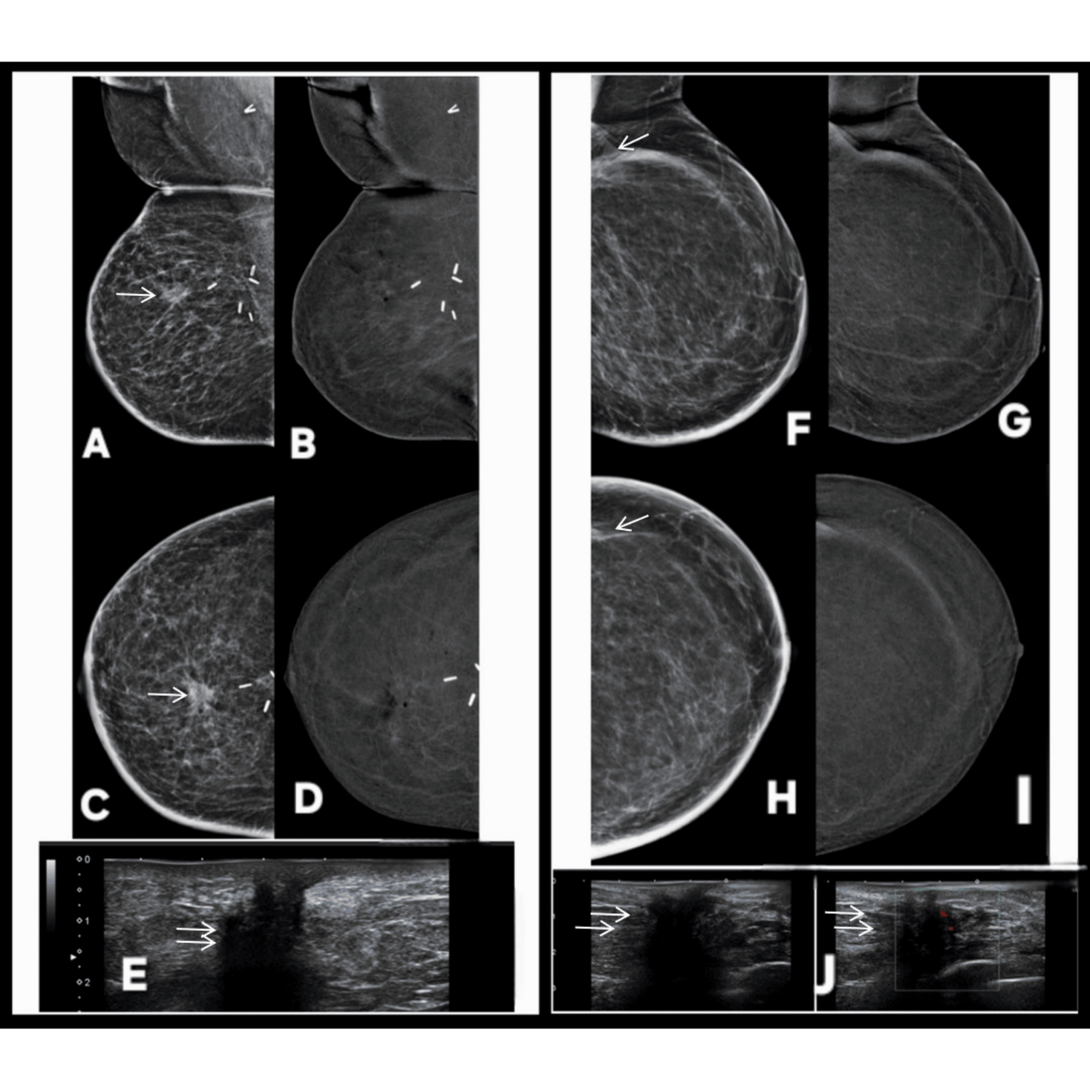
Contrast-enhanced mammography (CEM) as a problem-solving tool in post-surgical architectural distortion: two cases. Described abnormality is annotated by white arrows in all radiological images. Patient ε (Panels A–E): A 50-year-old woman, a three-year post–breast-conserving surgery case for invasive lobular carcinoma, presented for routine surveillance with no available prior imaging for comparison. Digital mammography (DM) in MLO and CC projections (A, C) demonstrates post-surgical clips and an ill-defined area of architectural distortion in the upper inner quadrant (white arrows). Given the oncologic history and absence of priors, the finding was categorized as BI-RADS 3. CEM (B, D) shows complete absence of contrast enhancement at the site of mammographic concern, effectively downgrading the abnormality to BI-RADS 2. Targeted grayscale ultrasound (E) reveals ill-defined posterior acoustic shadowing deep to surgical scar, initially appearing suspicious. However, due to non-enhancing CEM, the finding was reclassified as likely benign post surgical change. At six-month follow-up, stable sonographic appearance is seen, confirming benignity. Patient ζ (Panels F–J): a 55-year-old woman, six-month post–breast-conserving surgery case, was undergoing early routine follow-up. DM images (F, H) show ill-defined area of architectural distortion in the upper outer quadrant (white arrows), suspicious in appearance (BI-RADS 4). Grayscale ultrasound (J) shows a poorly marginated hypoechoic area without definable mass, likewise requiring additional evaluation. CEM (G, I), however, shows no enhancing mass or non-mass lesion, indicating absence of active pathological enhancement and supporting a benign postoperative etiology (BI-RADS 2). At six months, findings remained stable, corroborating the benign interpretation. In both cases, CEM provided critical diagnostic clarification when DM and ultrasound demonstrated architectural distortion with suspicious or indeterminate morphology. Lack of enhancement on CEM allowed confident downgrading preventing unnecessary biopsies. MLO: mediolateral oblique; CC: craniocaudal; BI-RADS: Breast Imaging Reporting and Data System
